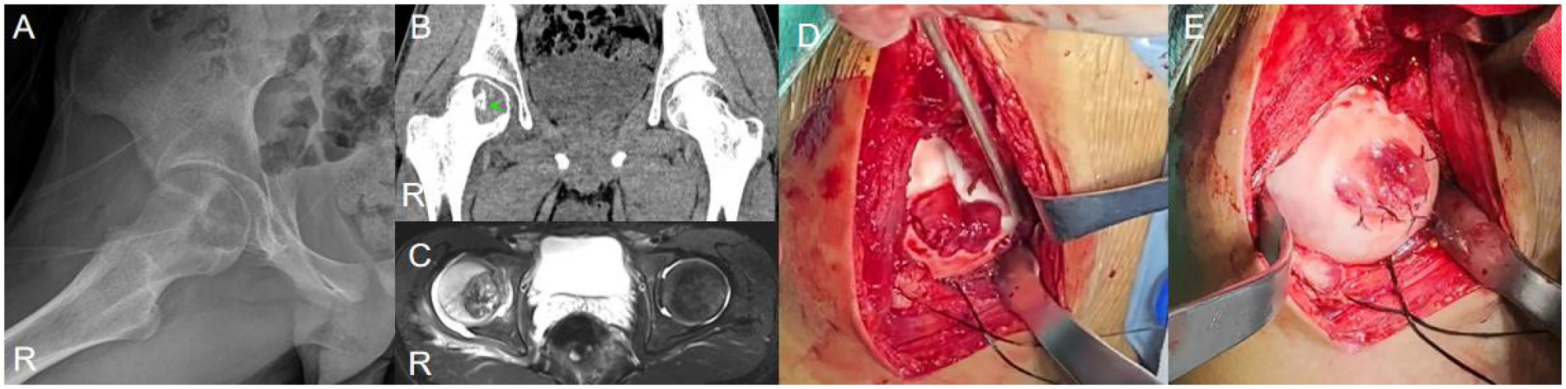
Case 1: (A) preoperative x-ray showed an isolated eccentric osteolytic lesion in the right femoral head. (B) CT (soft tissue window): Calcified tissue visible (green arrow). (C) MRI (T2WI-STIR): High signal in the femoral head on the tumor side, with irregular signals within the tumor. (D) Dislocation revealed joint cartilage destruction and joint surface collapse and deformity. (E) The smooth autologous iliac cortical bone was used for femoral head osteoplasty, and the damaged articular cartilage was sutured and repaired to flatten the joint surface.
